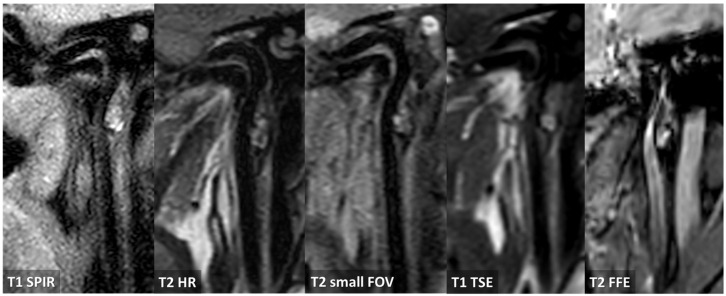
Vessel wall MRI with dedicated study of the prepetrous segment of the right ICA in oblique planes. Several sequences were used on the same segment, confirming the presence of a complicated atheroma with an intraplaque hemorrhage and a positive remodeling of the vessel wall.
